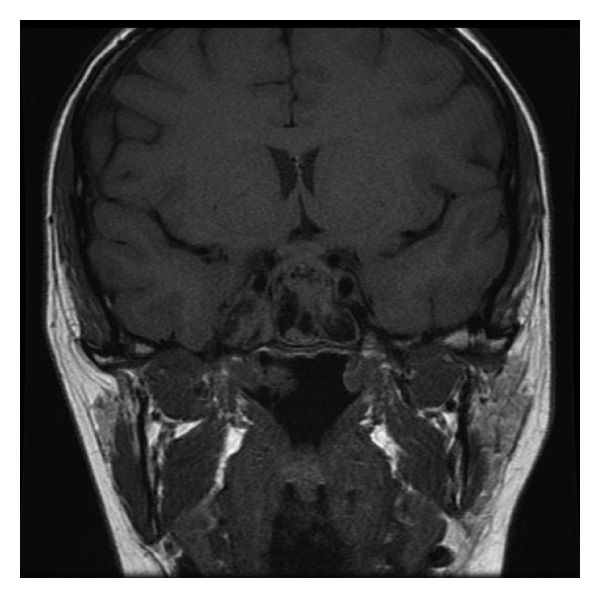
Coronal MRI image showing expanded sphenoid sinus with a suspected mucocele.
